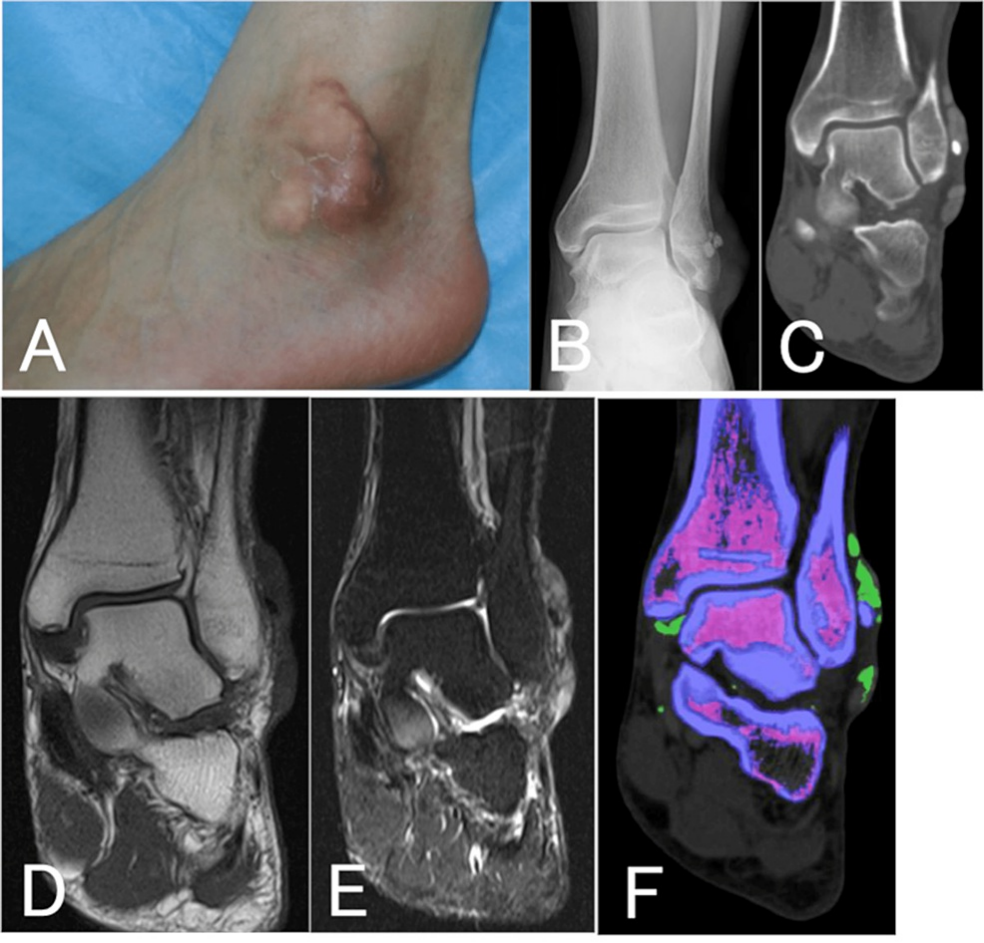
Case three: clinical and radiological findings. A: Initial examination revealed a mass in the left lateral malleolus. B, C: Radiographic and computed tomography images showing obvious calcification in the lateral malleolus with mass lesions above and below. D, E: T1-weighted (D) and T2-weighted (E) magnetic resonance images showing a mass-like lesion with a heterogeneous signal in the lateral malleolus. F: Dual-energy computed tomography scan with the gout nodule depicted in green.
